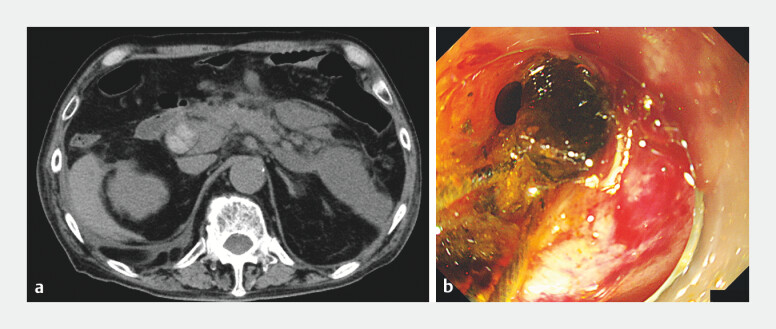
Computed tomography (CT) and endoscopic management of common bile duct (CBD) stones. Procedural sequence of CBD stone management during single-balloon enteroscopy in the present case.
